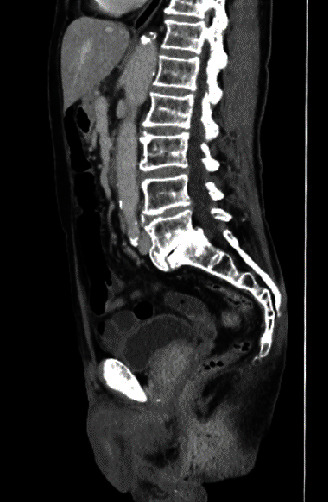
Contrast-enhanced abdominal CT scan. Sagittal section. Enhanced abdominal CT shows a contrast effect on the perianal skin in the early phase.
